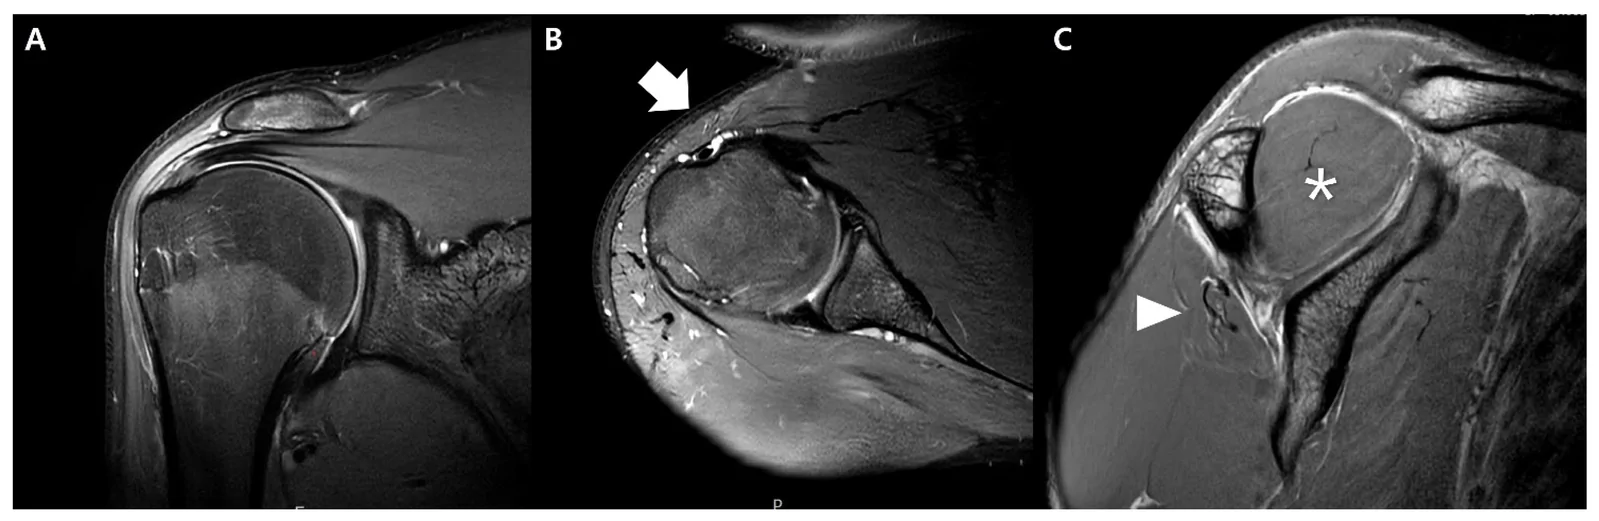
Magnetic resonance imaging findings of the right shoulder. (A) Coronal T2-weighted fat-suppressed image showing no definite rotator cuff tear, labral tear, paralabral cyst or other space-occupying lesion. (B) Axial T2-weighted fat-suppressed image demonstrating atrophy of the deltoid muscle, particularly in the anterior portion (arrow), with associated signal change and volume loss of the infraspinatus muscle. (C) Sagittal T1-weighted image showing fatty degeneration and atrophy of the infraspinatus muscle (arrowhead) with relative preservation of the supraspinatus muscle (asterisk).
